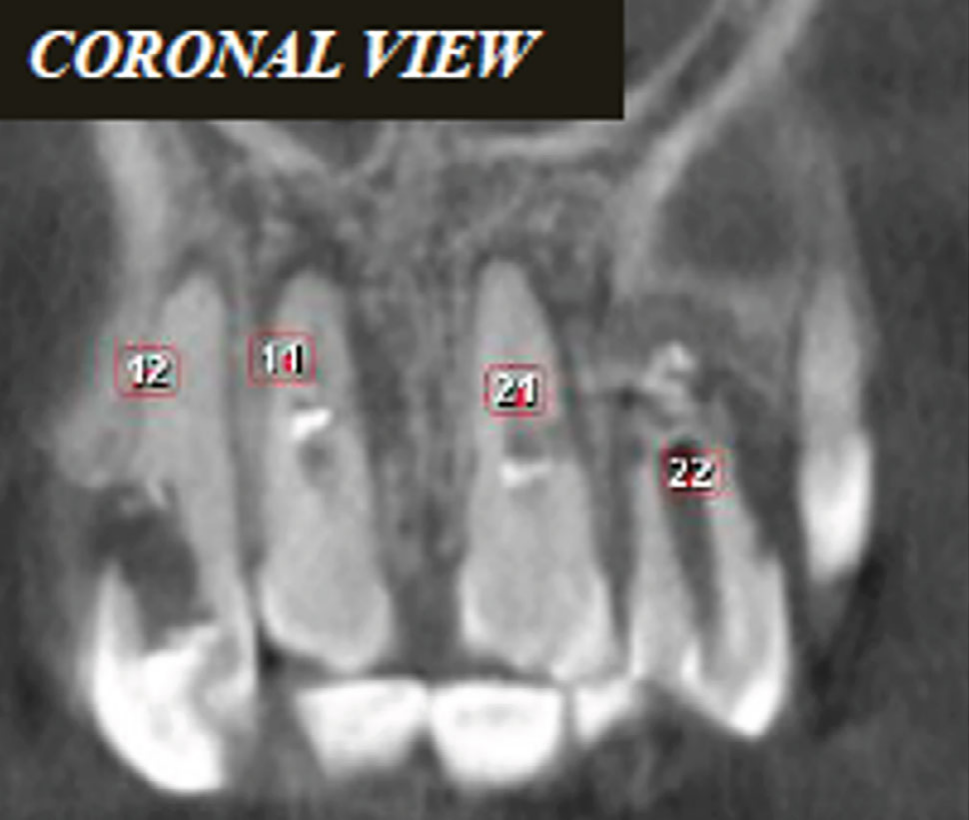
CBCT image showing Tooth Numbers 12, 11, 21 and 22.
